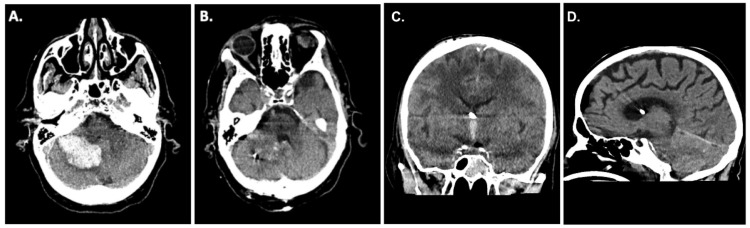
(A) Preoperative CT scan with the diagnosis of a spontaneous cerebellar hemorrhage on the right side. (B) CT scan after hematoma evacuation and insertion of a Spiegelberg 3-PN sensor in the cerebellar parenchyma on the right side. (C) Postoperative CT scan showing the correct location of the combined external ventricular drain-ICP probe in the right ventricle. (D) CT scan after hematoma evacuation showing no sign of transtentorial herniation.
